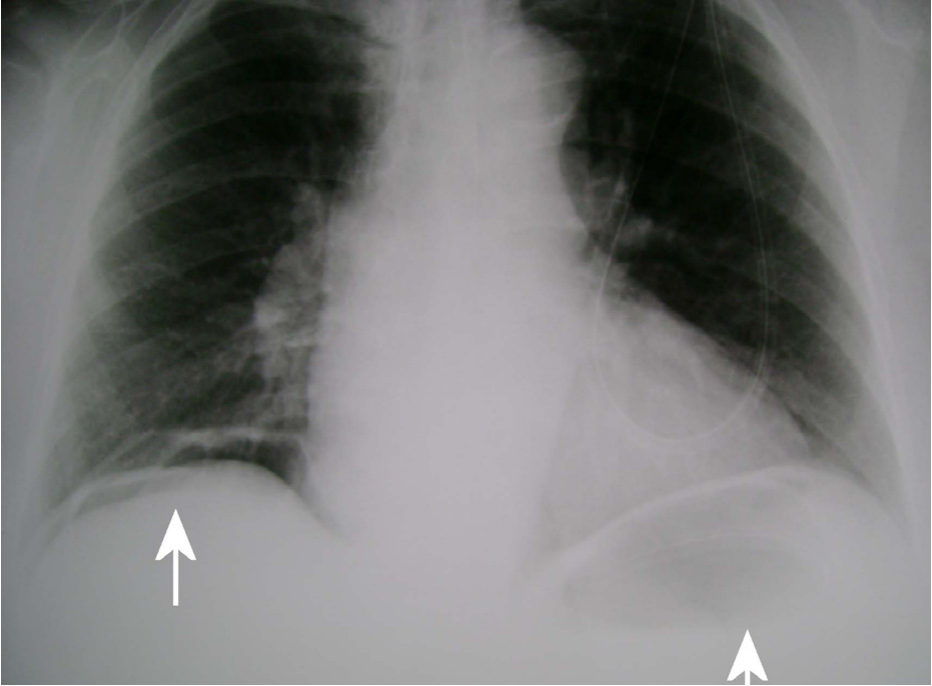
Upright posteroanterior chest radiograph after insufflating air into the stomach. The free subdiaphragmatic air has slightly increased in size bilaterally compared with Figure 1 (*white arrows*).
